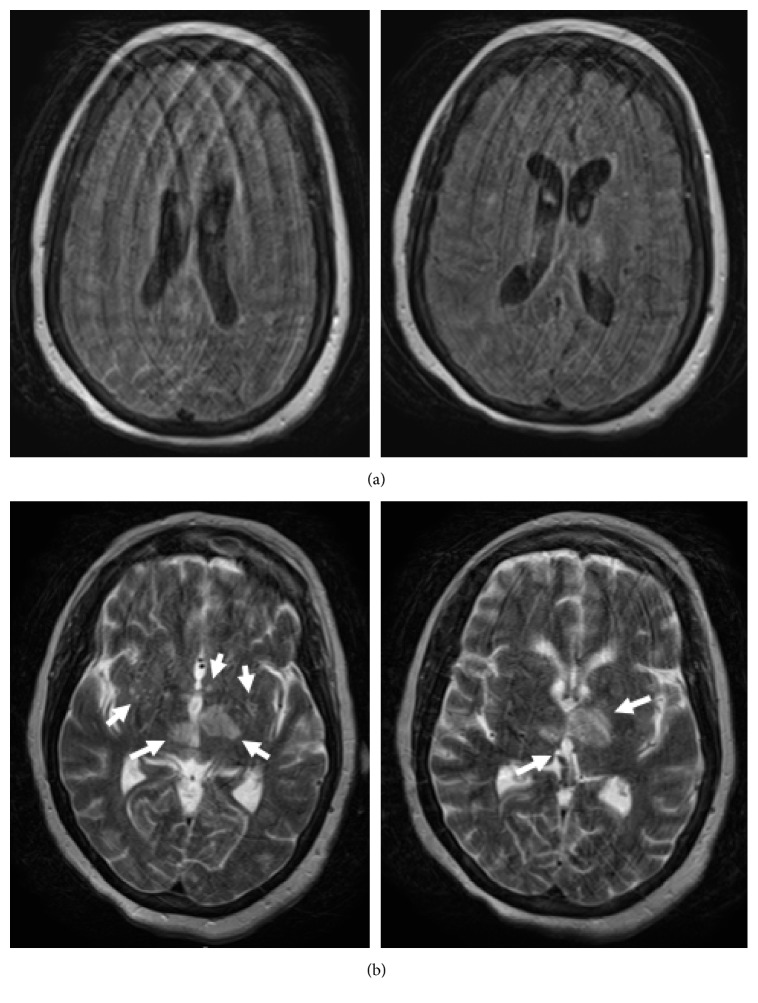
Repeat MRI of the brain on hospital day 10. (a) There is much less FLAIR enhancement of the ventricles, consistent with improving infection. (b) T2 weighted images showing several new infarcts within the bilateral thalami, basal ganglia, and right parietal lobe (arrows). Also visible are older, evolving infarcts.
